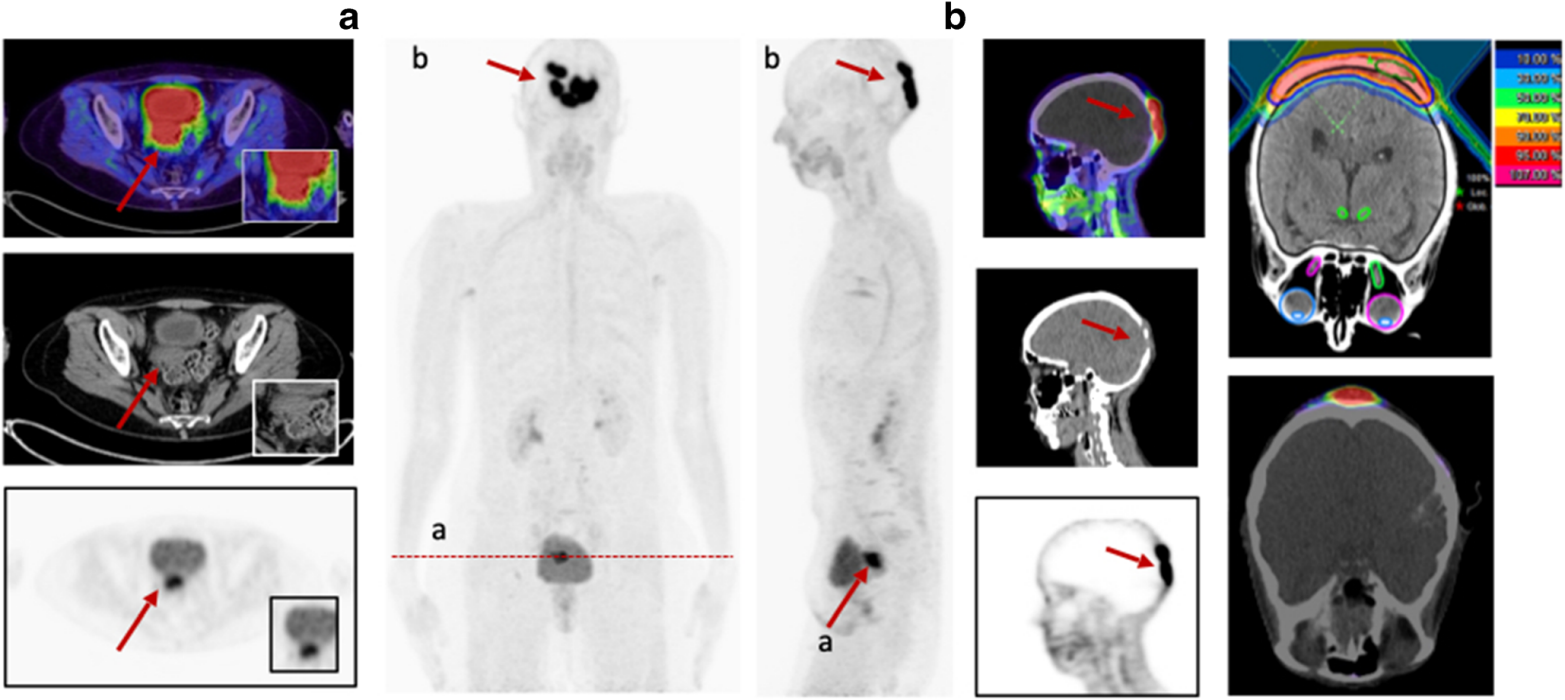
A 58-year-old female patient presented with cervical cancer for pre-radiotherapeutic staging due to a skull metastasis using ^68^Ga-FAPI-PET/CT. The investigation showed a local relapse with a SUVmax of 14.4. Furthermore, the skull metastasis presented a strong FAPI uptake with a SUVmax of 32.3 enabling precise delineation and radiotherapy planning
